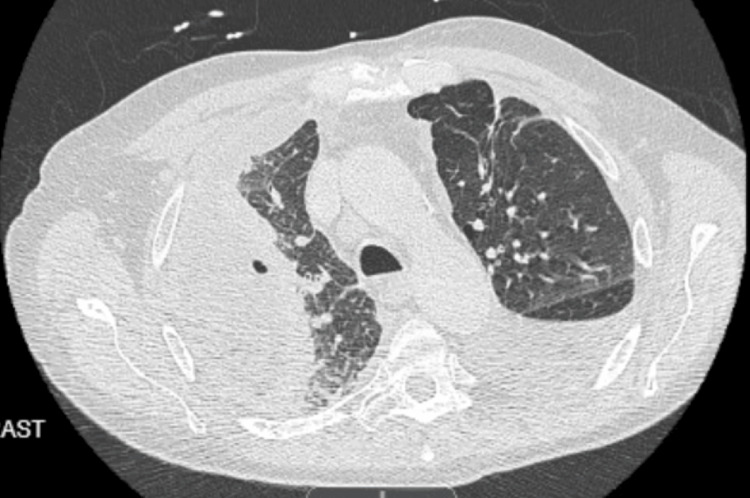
CT chest. Axial view Showing a large, loculated right pleural effusion with the right chest drain in the appropriate position. Air pockets in the fluid could be related to the presence of the chest drain or could represent an infectious process. There is dense compressive atelectasis of the right lung and moderate left pleural effusion.
